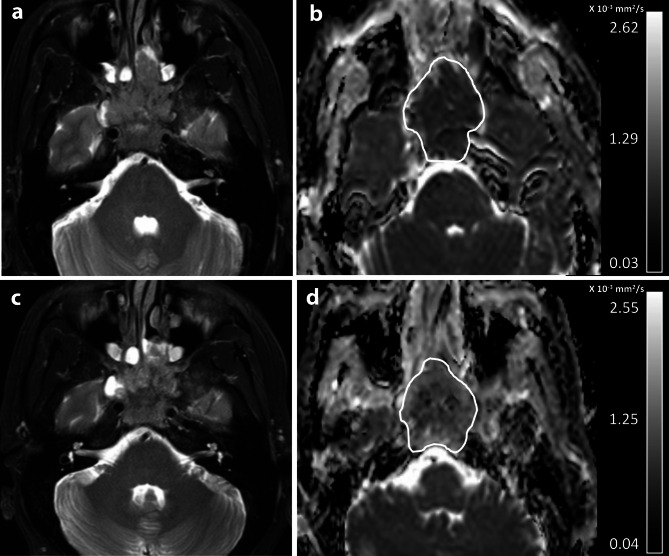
Pre-treatment (a-b) and post-IC (c-d) MRIs of a patient with stage T4 NPC that had no recurrence 36 months after treatment. The axial images comprise T2-weighted fat-suppressed images (a and c) and ADC maps (b and d). The mean ADC_pre_ and ADC_post−IC_ extracted from the primary NPC (white contours) on the ADC maps were 0.81 × 10^− 3^ mm^2^/s, and 1.14 × 10^− 3^ mm^2^/s, respectively and the ΔADC% was 40.7%. The good long-term outcome was predicted by a high percentage increase in mean ADC value (ΔADC% of ≥ 34.2%) on the post-IC DWI compared with that on the pre-treatment DWI.
